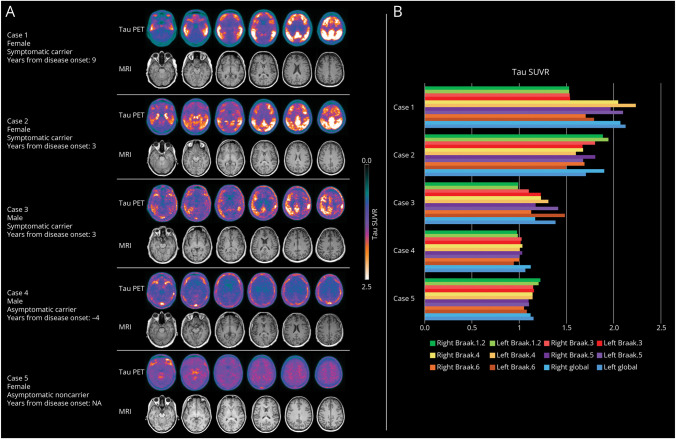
Tau and Neurodegeneration Patterns In Panel A, axial images of ^18^F-flortaucipir and MRI T1 images are shown for each study participant. ^18^F-flortaucipir PET and MRI T1 images were acquired the same day or within 2 days. The color bar shows SUVR ranges. In Panel B, the histogram shows the regional SUVRs for each participant. SUVR = standardized uptake value ratio.
